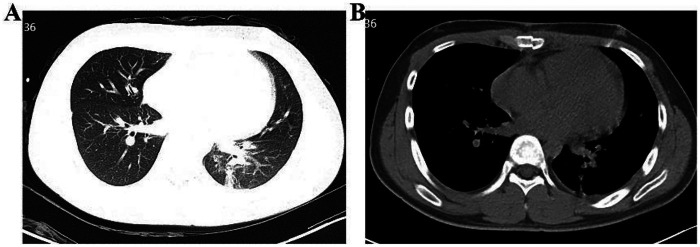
Postoperative non-contrast chest CT demonstrating lung re-expansion without evidence of infection. (A) Lung window; (B) Mediastinal Window.
